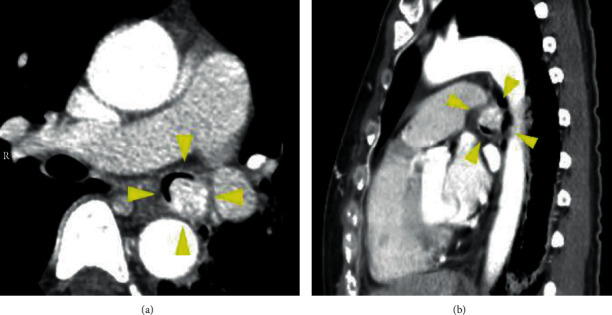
Transverse (a) and sagittal view (b) of a chest CT, taken after delivery, revealed a bronchial tumor in the left main bronchus without invasion to surrounding organs (yellow arrowheads).
